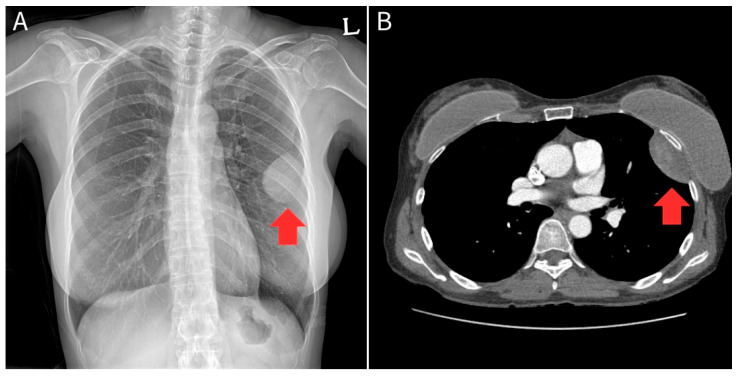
Preoperative radiological findings of the patient in Case 2. (A) The posteroanterior chest radiograph reveals a well-defined, rounded radiopacity in the left middle lung zone. (B) The chest computed tomography scan demonstrates a 5.3 cm, well-circumscribed, solid mass in the left hemithorax. The tumor forms an obtuse angle with the adjacent mediastinal pleura near the lingular segment, indicating its pleural origin. The mass appears homogeneous without evidence of calcification, necrosis, or invasion into surrounding structures.
